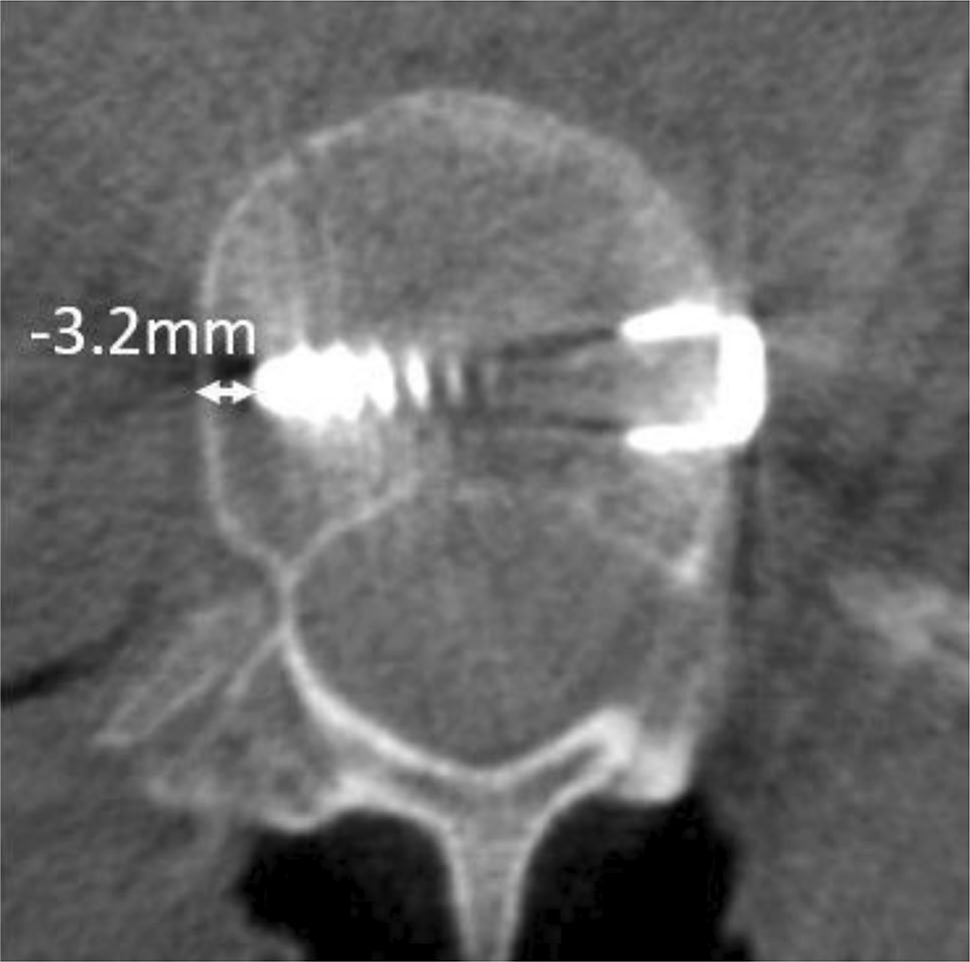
Axial CT image showing measurement of the distance from the tip of a screw to the far cortex of the vertebral body
